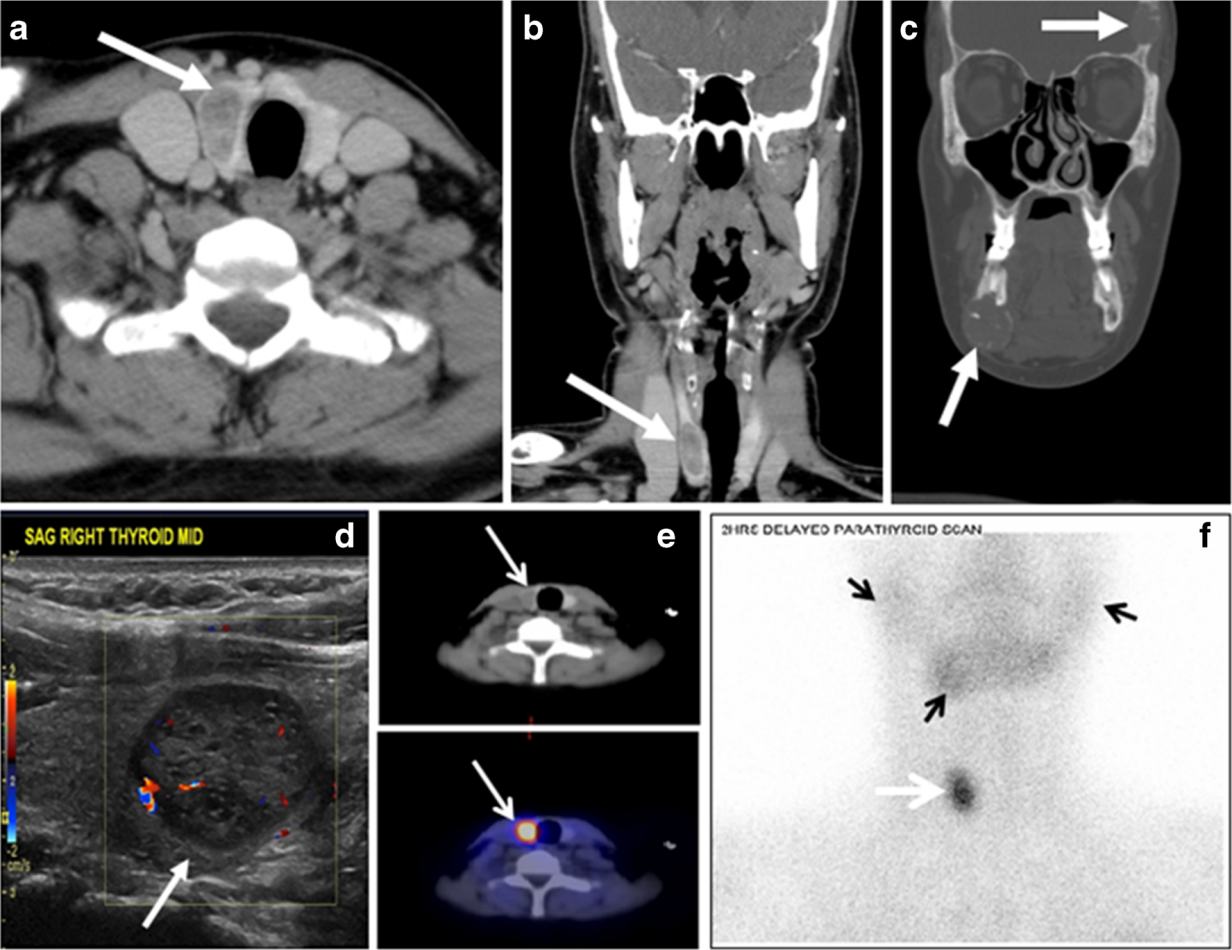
A 26-year-old male patient with elevated serum parathyroid hormones and calcium secondary to intra-thyroid parathyroid adenoma. a, b Enhanced axial and coronal CT scan of the neck demonstrate a well-defined hypodense right thyroid nodule (*white arrows*). c Bone window coronal CT scan shows lytic expansile lesions at the right mandible and left frontal bone (*white arrows*). d Transverse colour Doppler ultrasound of the neck demonstrates a well-defined, heterogonous, predominantly hypoechoic right thyroid nodule measuring 2.7 cm, with mild increased vascularity and no internal micro-calcifications (*white arrow*). e, f Delayed anterior planar and fused SPECT/CT parathyroid Sestamibi scan at 2 hours demonstrate persistent focal activity in the right thyroid lobe (*white arrows*). Note the scattered mandibular/maxillary uptakes in planar image representing the known brown tumours
